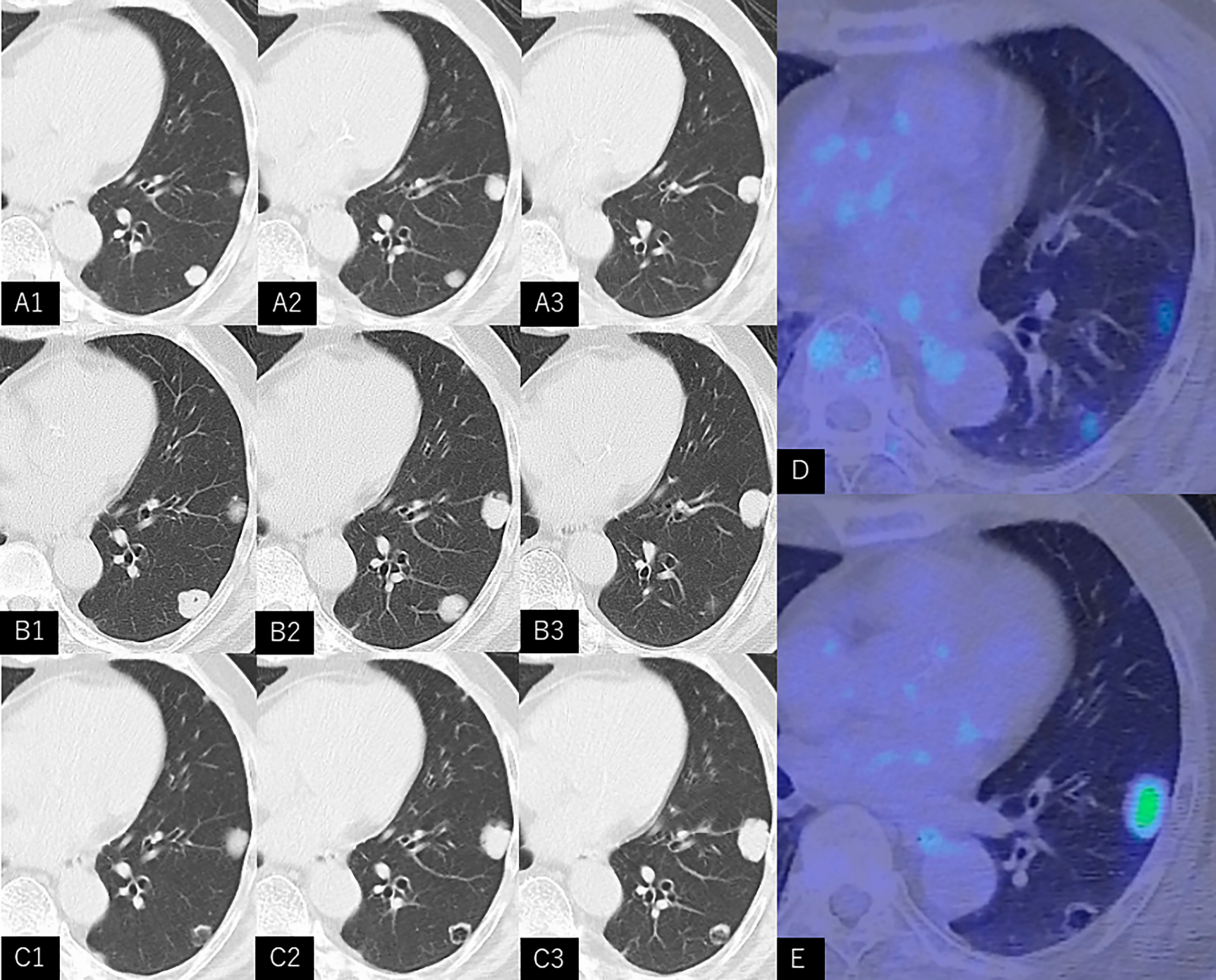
Chest computed tomography (CT) scan (A1–A3, B1–B3, and C1–C3) and fluorodeoxyglucose‐ positron emission tomography (FDG‐PET) findings (D, E). Two years before referral to our institution, a chest CT revealed nodular opacities in S6 and S8 of the left lower lobe (A1–A3). A PET scan showed FDG uptake within the nodules (SUVmax 2.34 for S6 and 2.40 for S8) (D). A follow‐up chest CT scan after 1 year showed that both nodules were enlarged (B1–B3). A chest CT scan after 2 years showed that the S8 nodule had enlarged in size; however, the S6 nodule had cavitated and decreased in size (C1–C3). A PET scan showed FDG uptake in the S8 nodule (SUVmax 4.26) but not in the S6 nodule (E).
